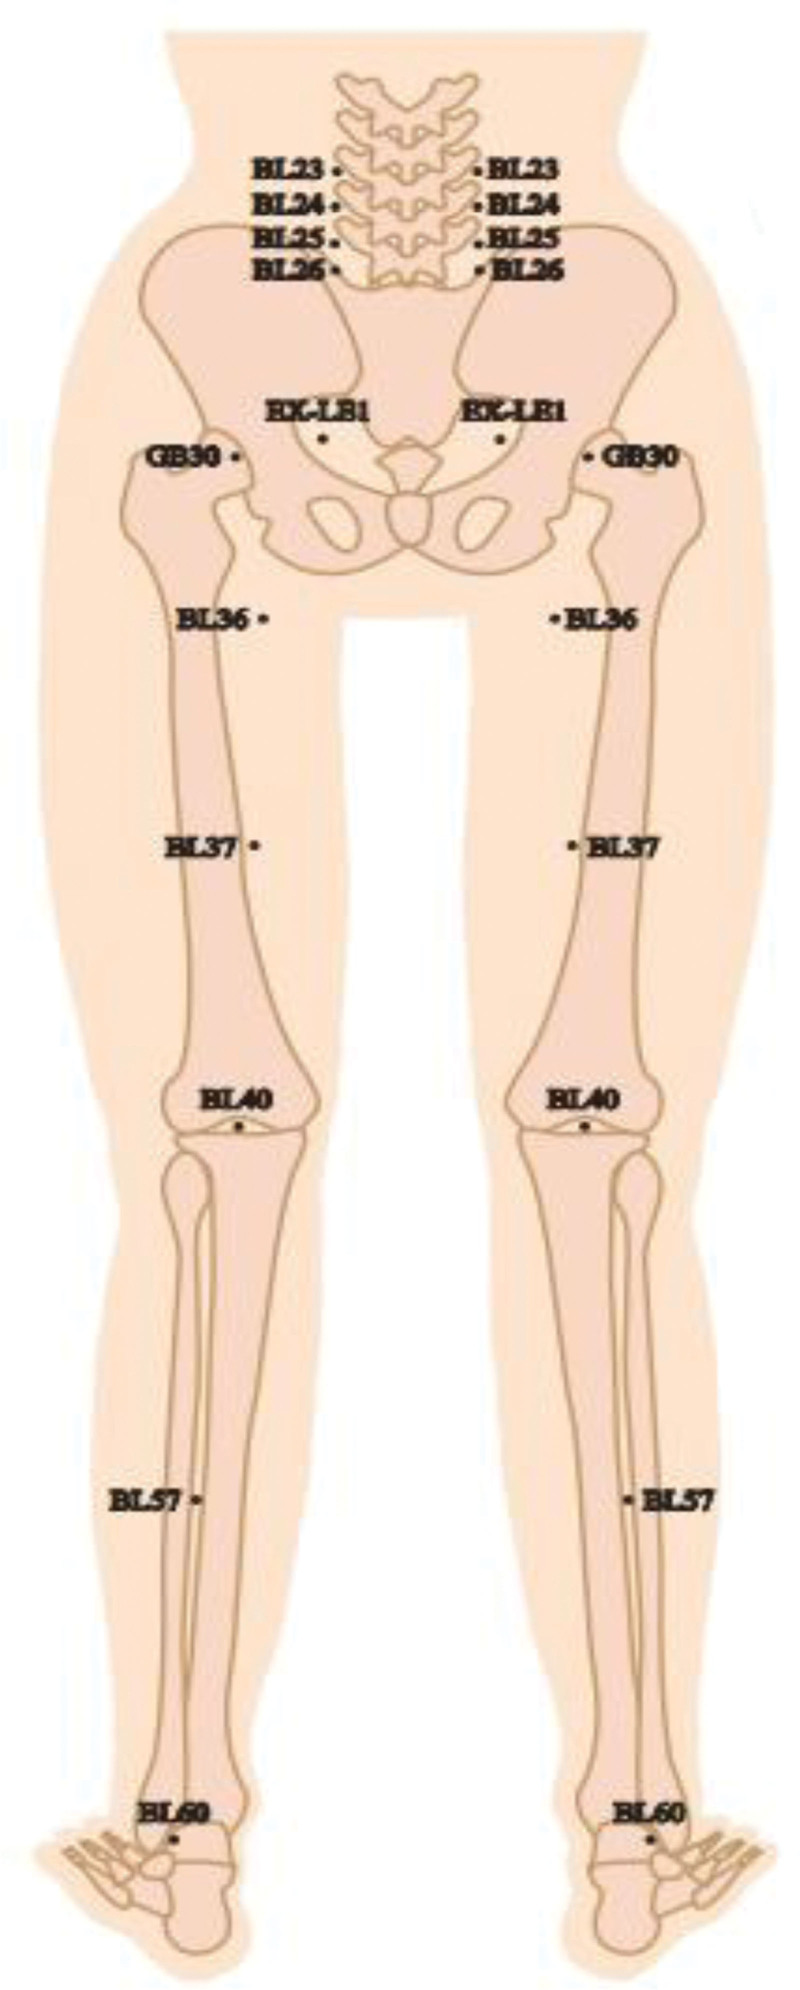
5 years after follow-up, the patient lumbar pain and lower extremity pain did not recur, and the lumbar MRI scan was reviewed on October 11, 2022: lumbar degeneration, degenerative bulging of L4/5 and L5/S1 discs, and endplate inflammation of L5 and S1 vertebrae (Fig. 3A,B).
